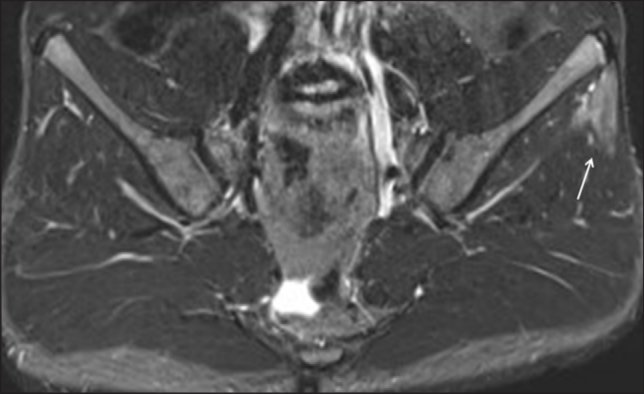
Semicoronal STIR MR image of the SI joint in a 15-year-old boy with arthralgias (ERA-negative) shows edema at the origin of the gluteus medius muscle at the iliac crest representing enthesitis.
